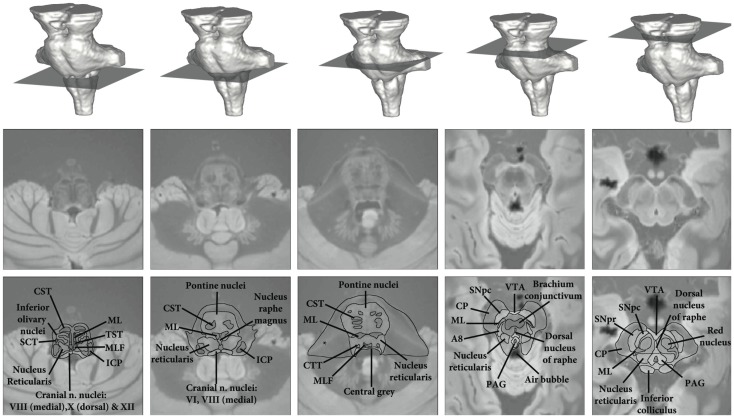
High resolution *ex vivo* combined MT T2* MRI with anatomical annotations for reference. Figure adapted from Lambert et al. (2013) with permission from Elsevier. Abbreviations: A8, dopaminergic center (approximate location), CP, cerebral peduncle (anterior to posterior: consisting of frontopontine, corticonuclear, corticospinal, and parietotemporal pontine tracts); CST, corticospinal tract; CTT, central tegmental tract; ICP, inferior cerebellar peduncle; ML, medial lemniscus; MLF, medial longitudinal fasciculus; PAG, periaqueductal gray; SCT, spinocerebellar tract; SNpc, substantia nigra pars compacta; SNpr, substantia nigra pars reticulata; TST, tectospinal tract; VTA, ventral tegmental area. *Artifact due to fixation.
